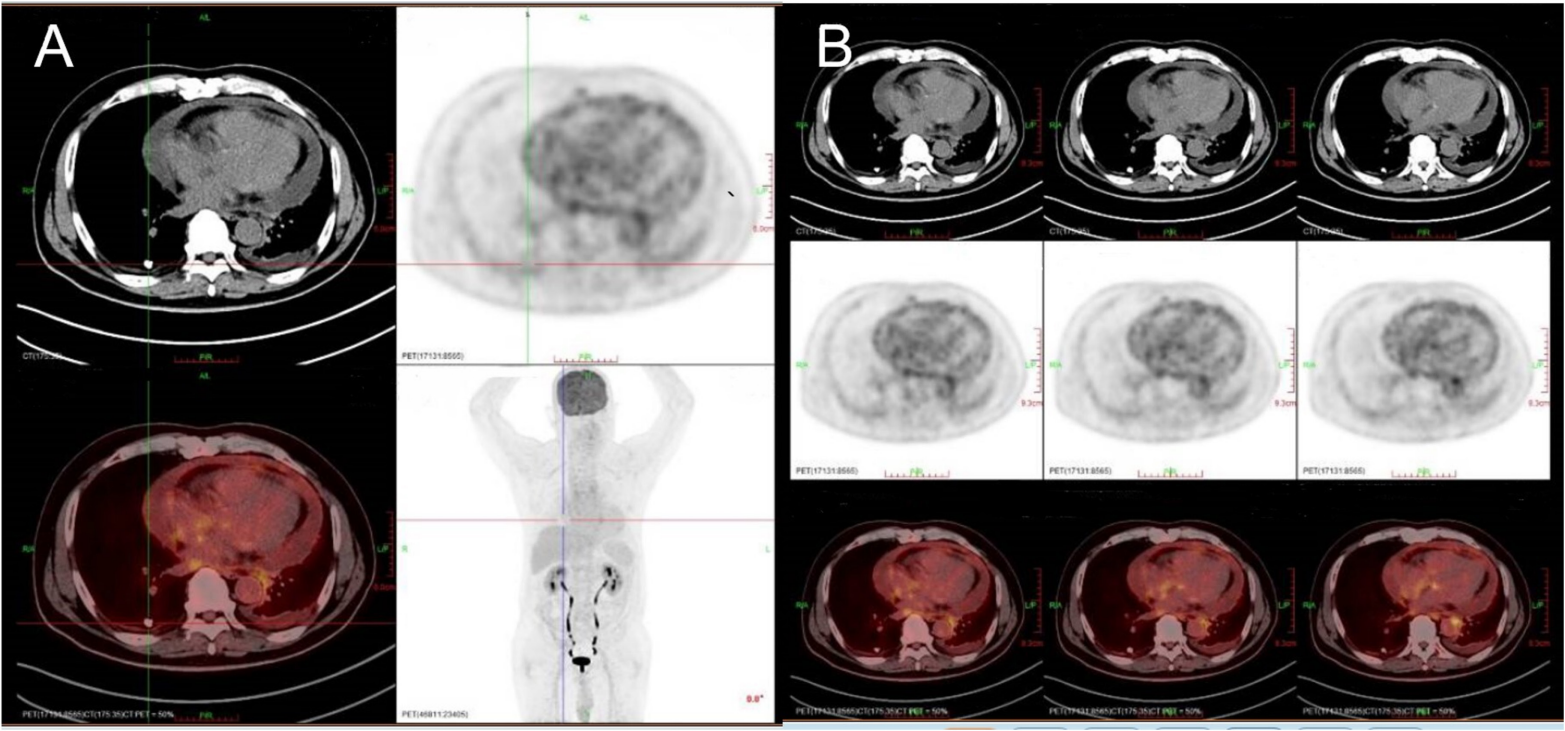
(A,B) PET/CT showed pericardial effusion with slightly increased metabolism along the pericardium.
